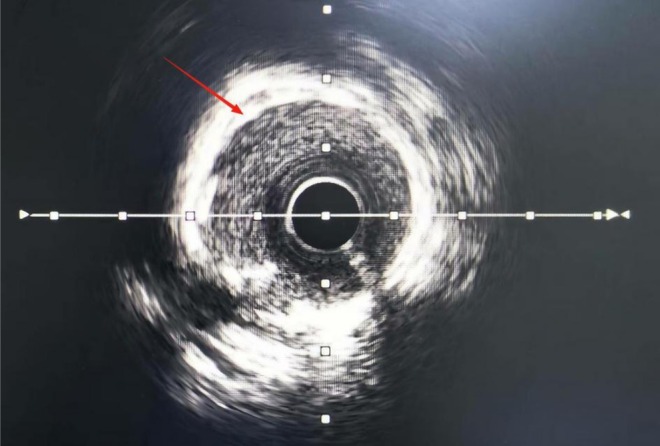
Intravascular ultrasound image demonstrating good stent expansion and vessel wall apposition following implantation.
